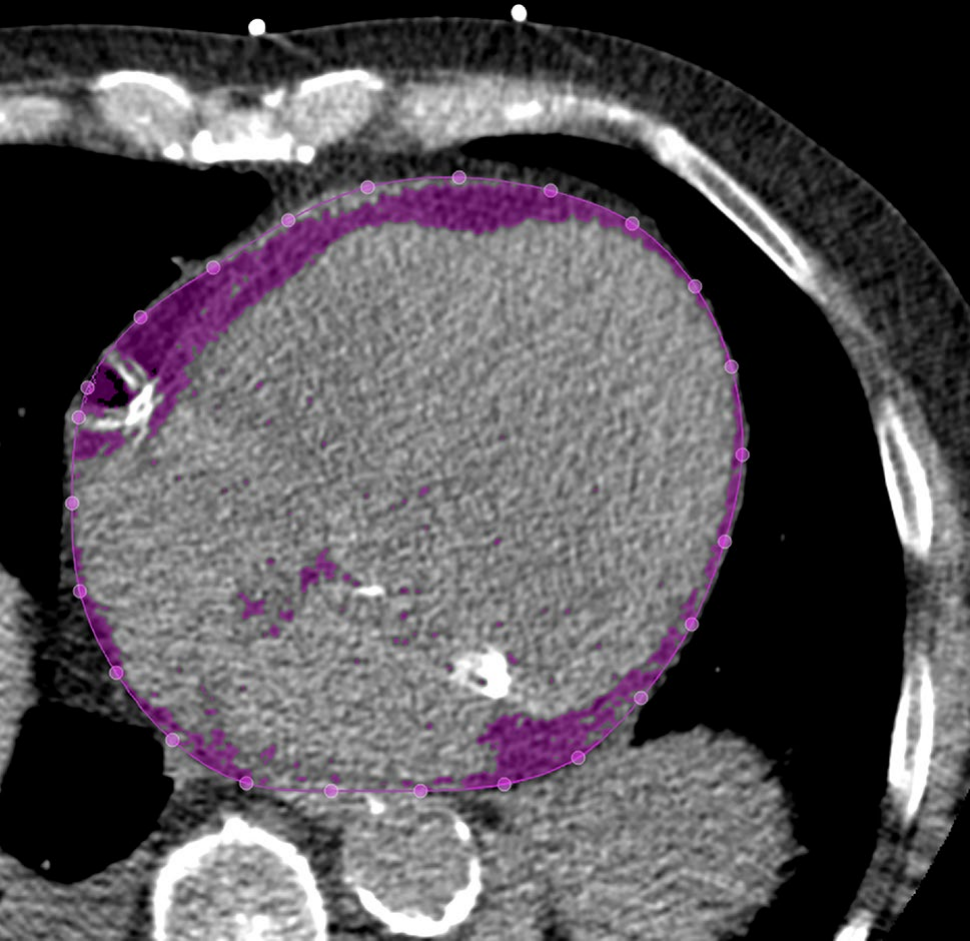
Example of an axial CT slice of the heart, with EFT shown in purple after automatic tracing of the pericardial border by the fully automated QFAT software. *CT* computed tomography, *EFT* epicardial fat tissue
